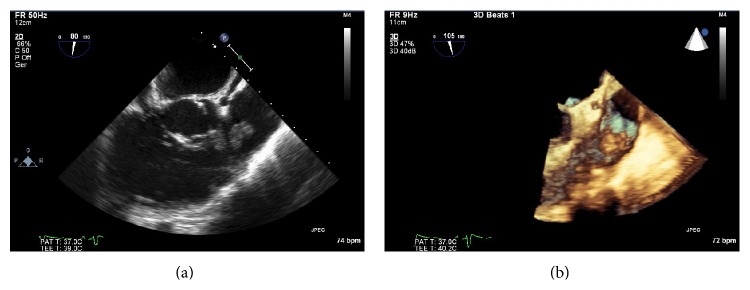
Transesophageal echocardiogram with a 2.4 × 1.8 cm echodense mobile mass seen on the pulmonic valve consistent with large vegetation in 2D and 3D views.
